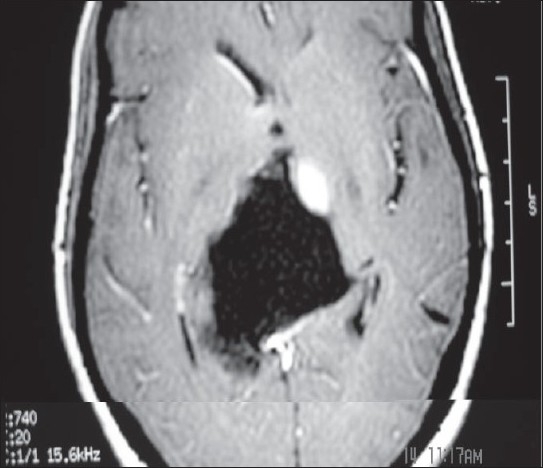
Preoperative T1-weighted axial magnetic resonance imaging showing a giant epidermoid cyst in the quadrigeminal cistern
